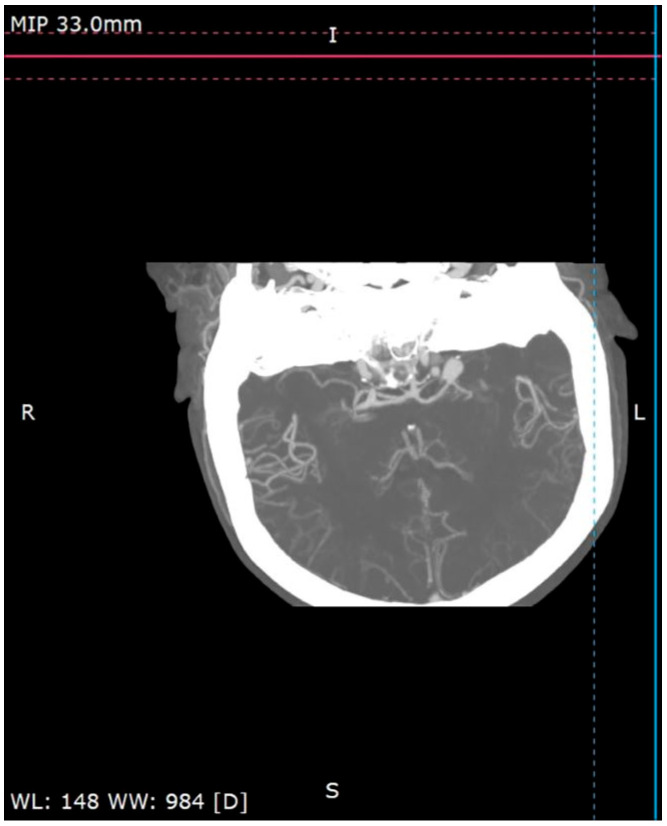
Cerebral CT angiography.
